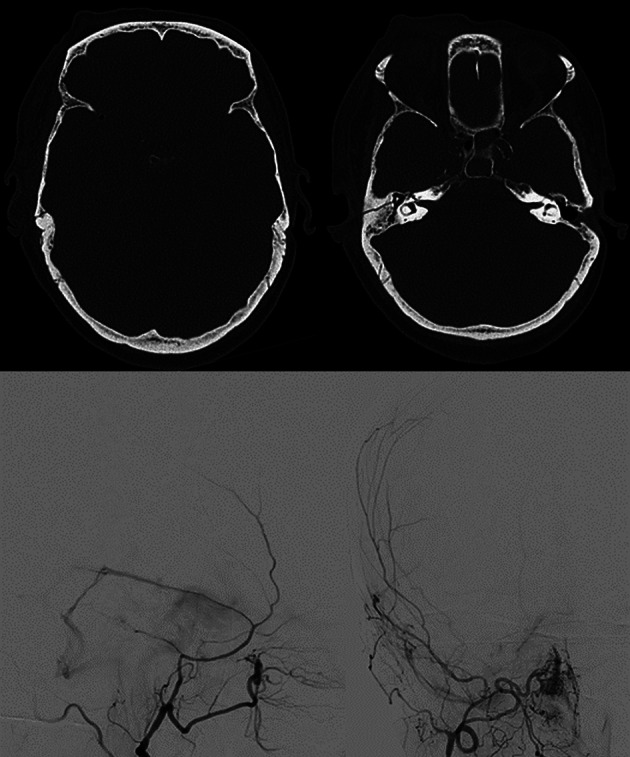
Blunt trauma in a patient in the early eighth decade of life. CT scan (top row) shows a non-otic longitudinal fracture of the right petrous bone. DSA (bottom row) performed while injecting the right external carotid artery territories, demonstrates the presence of a post-traumatic dural AVF between the posterior branch of the middle meningeal artery and the ipsilateral transverse sinus (Borden type I)
